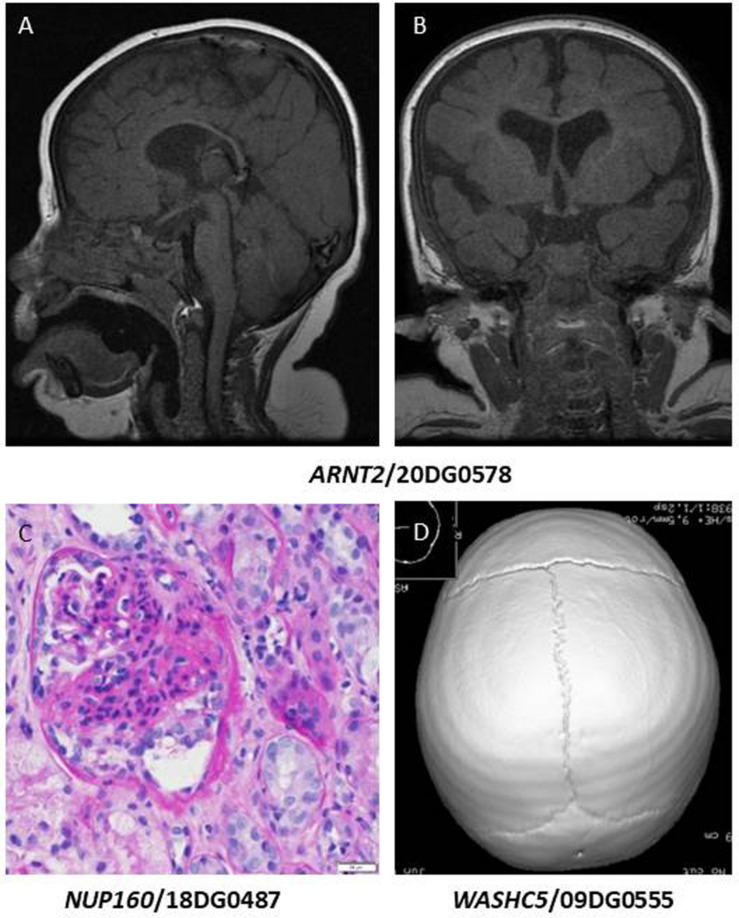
(A,B) Brain MRI images of the patient with homozygous *ARNT2* mutation showing thinning of the corpus callosum, brain atrophic changes, and hypoplasia of the pituitary gland with no demonstrable posterior lobe bright spot or pituitary stalk. (C) Renal biopsy of the patient with *NUP160* mutation showing segmental solidification of the glomerular tuft (classical segmental scar). (D) CT scan image of the patient with a variant in *WASHC5* showing partial premature closure of the lambdoid suture.
